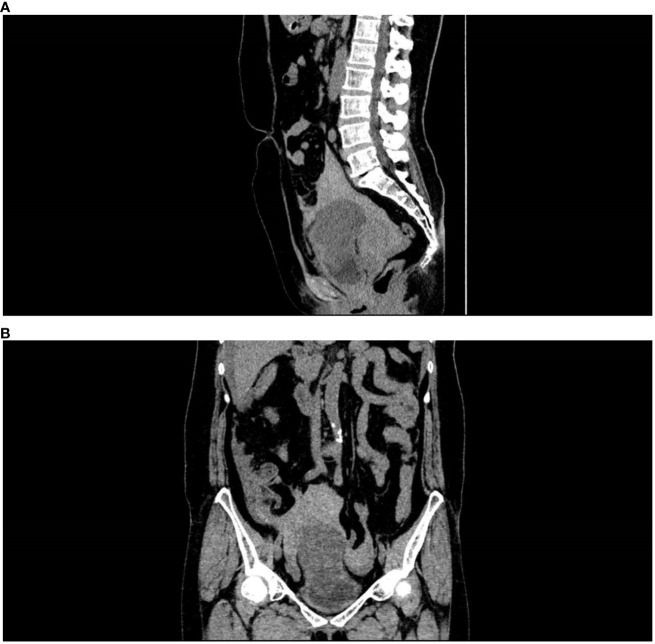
Enhanced CT scan shows pelvic hemorrhage. (A) Side view, (B) Front view.
